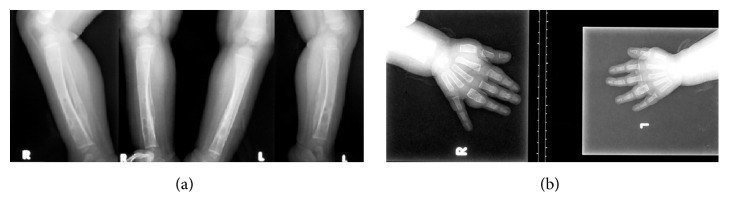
Disseminated Bacillus Calmette-Guérin (BCG) infection of the upper and lower extremities in an infant produces similar images (June–September 2012). (a) Bilateral lateral and anteroposterior conventional radiographs of the lower parts of both legs show multiple radiolucent cystic lytic areas of bone destruction with minimal periosteal reaction and bone widening in the distal diaphysis of tibia and fibula. (b) Conventional posteroanterior radiographs of both hands revealed periosteal elevation and multiple small lytic lesions with bone widening in the proximal phalanges of the fourth fingers bilaterally.
